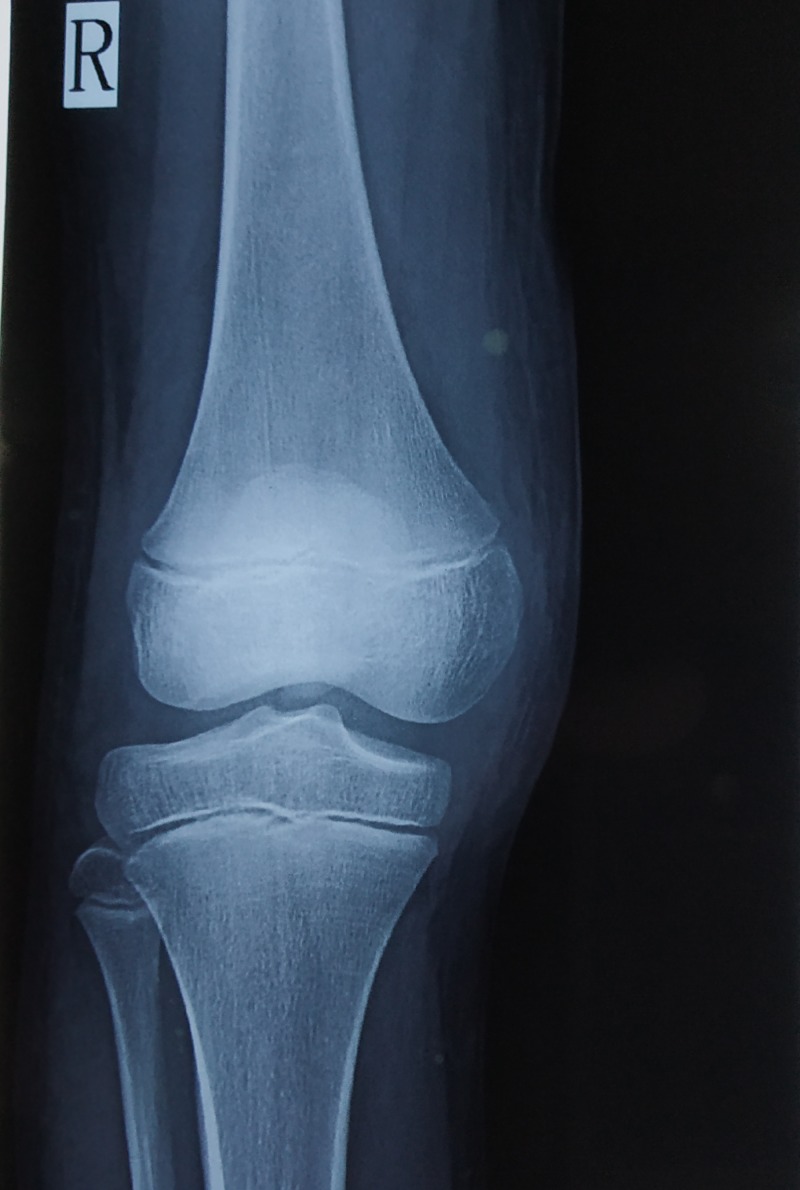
Post-operative X-ray of the right knee joint: anteroposterior view
